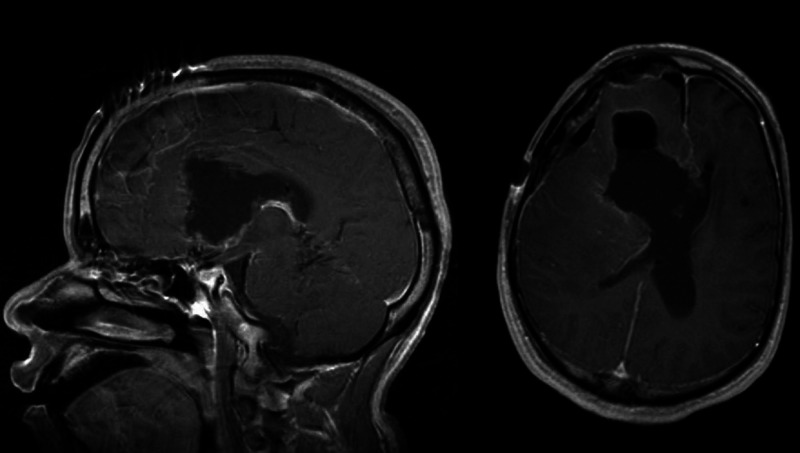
Postoperative sagittal and axial T1-weighted MRI with contrast, showing complete resection of the encapsulated hematoma and retained nonfunctioning shunt.
